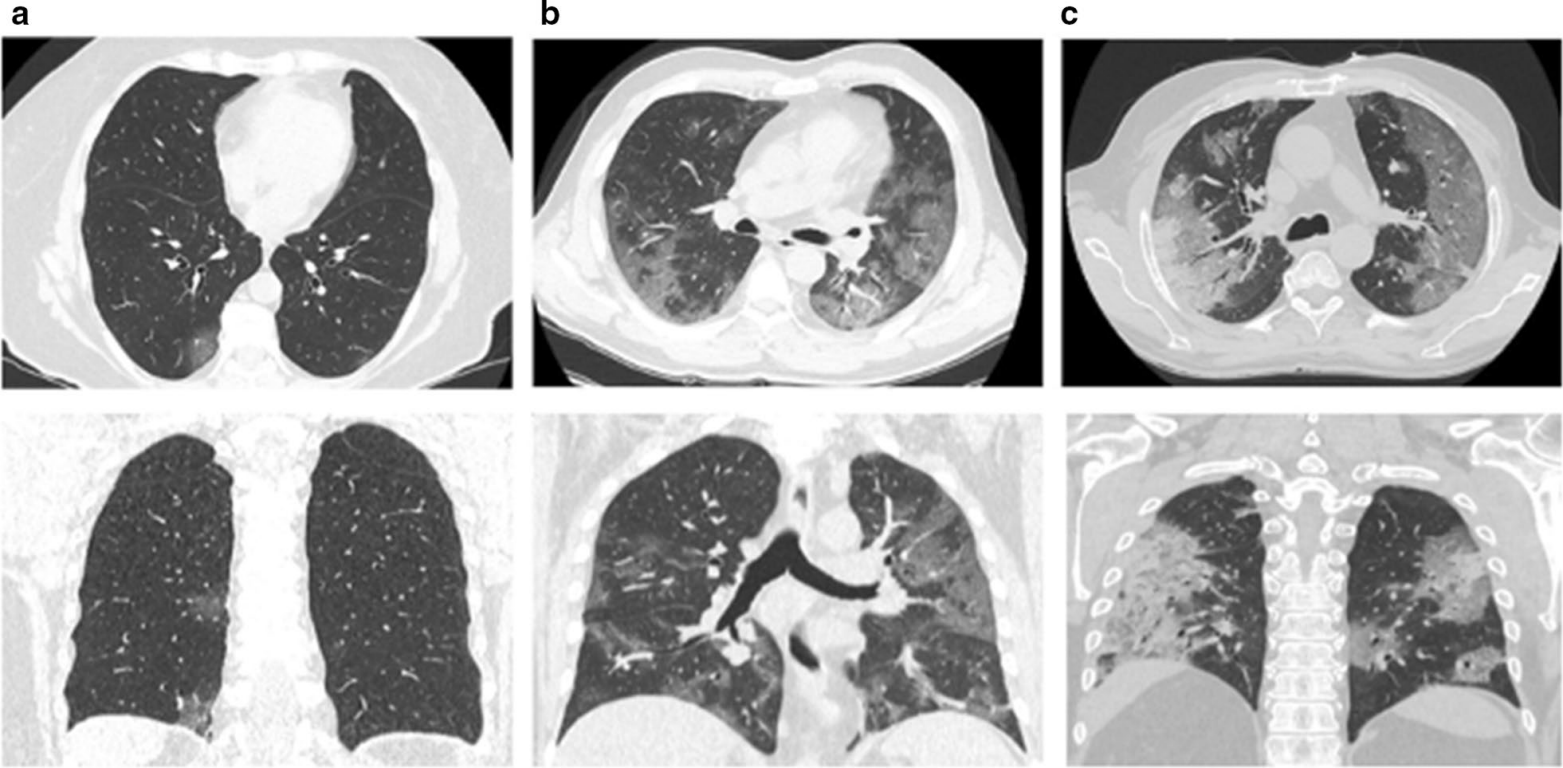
COVID-19 interstitial lung pneumonia radiologic images in patients with or without Low T3 syndrome. Representative CT images of different degrees of severity in a patient with normal FT3 value (a) and in two patients with low FT3 levels (b, c). a Minimal involvement of right inferior lobe. Two ground glass opacity in the subpleural space. b Moderate involvement of both lungs. Diffuse ground glass opacities, with peripheral distribution and subsegmental vessel enlargement. c Severe involvement of both lungs. Diffuse ground glass opacities, especially with peripheral distribution. Consolidations are also detected, mostly with peripheral distribution, in right upper lobe posterior segments. For each case both axial and coronal sections are shown
